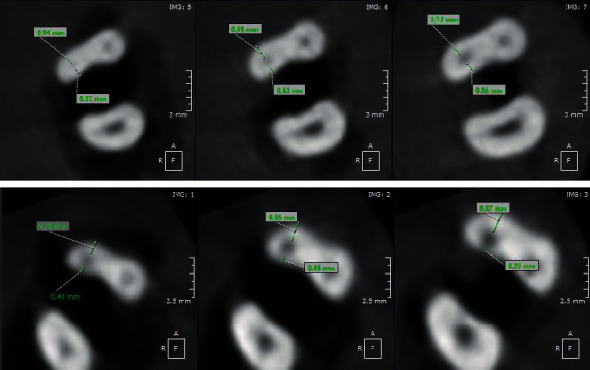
Typical CBCT images of samples.
